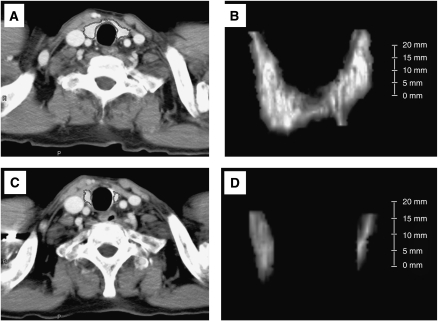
Measurement of thyroid gland volume using CT scan. A cross-sectional image (A) and three-dimensional (3D) reconstruction image (B) of the thyroid gland in CT scan with contrast at baseline in patient 5 (thyroid volume 7.8 ml). A cross-sectional image (C) and 3D reconstruction image (D) of the thyroid gland in the same patient 15 months after the start of sunitinib treatment (thyroid volume was 1.2 ml).
